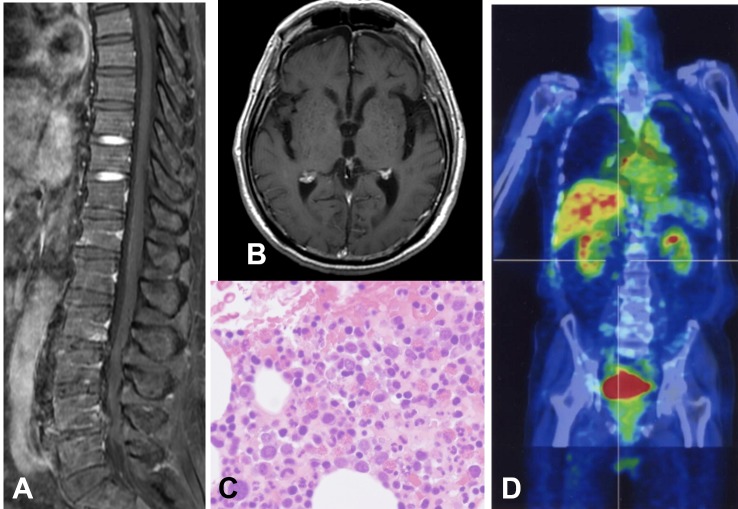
Other examinations Magnetic resonance imaging (MRI) of the lumbar spine and brain (A and B), bone marrow puncture (C) and ^18^F-fluorodeoxy-glucose positron emission tomography (18FDG-PET) of the whole body (D) showing no other primary lesion.
